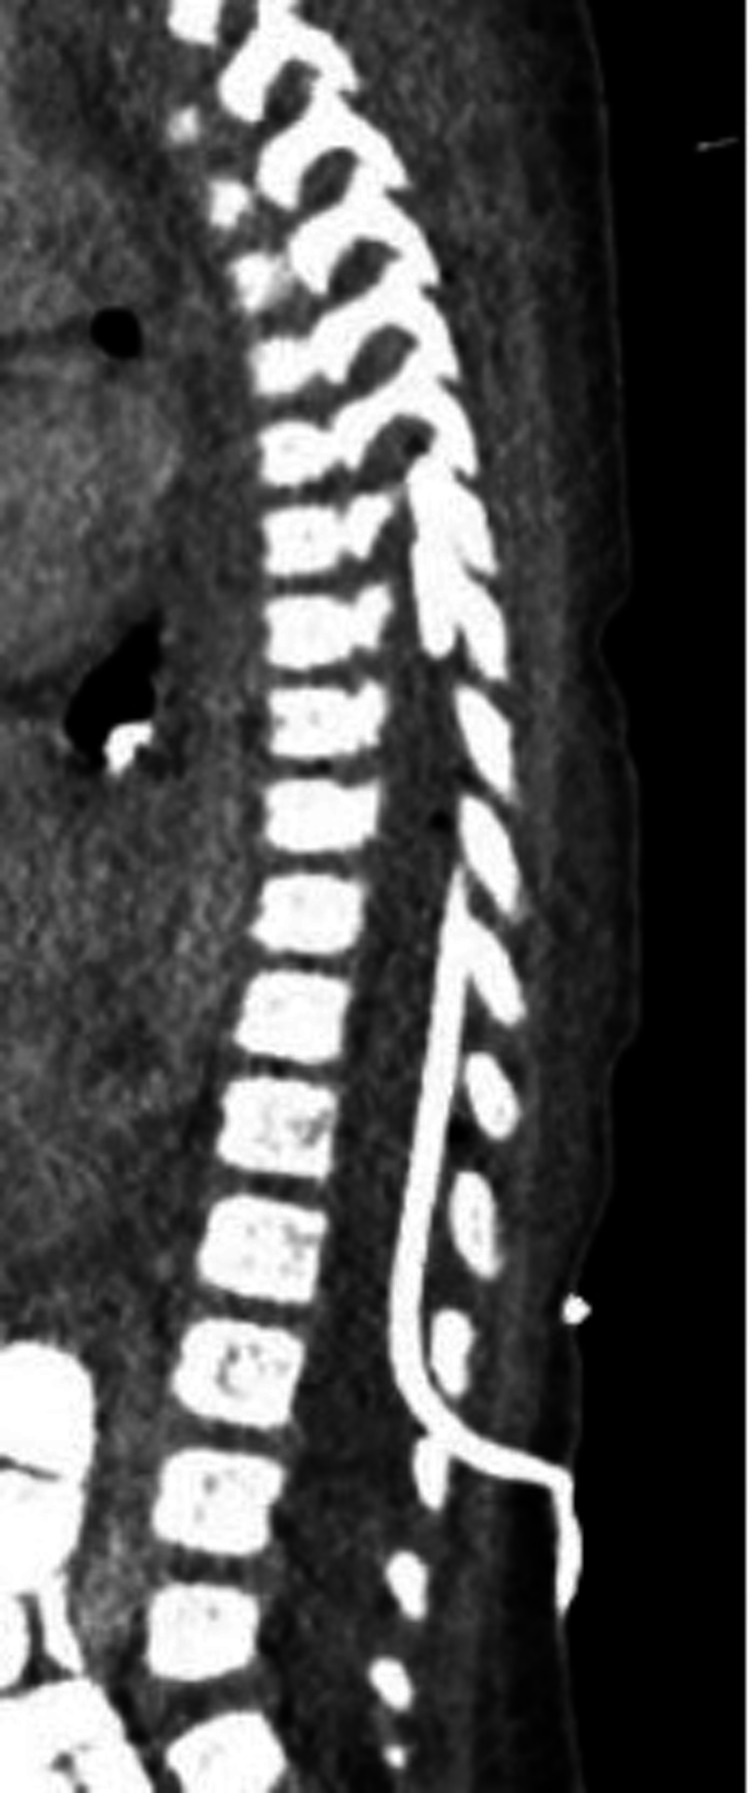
Postoperative CT demonstrating catheter in the epidural space. CT: computed tomography
